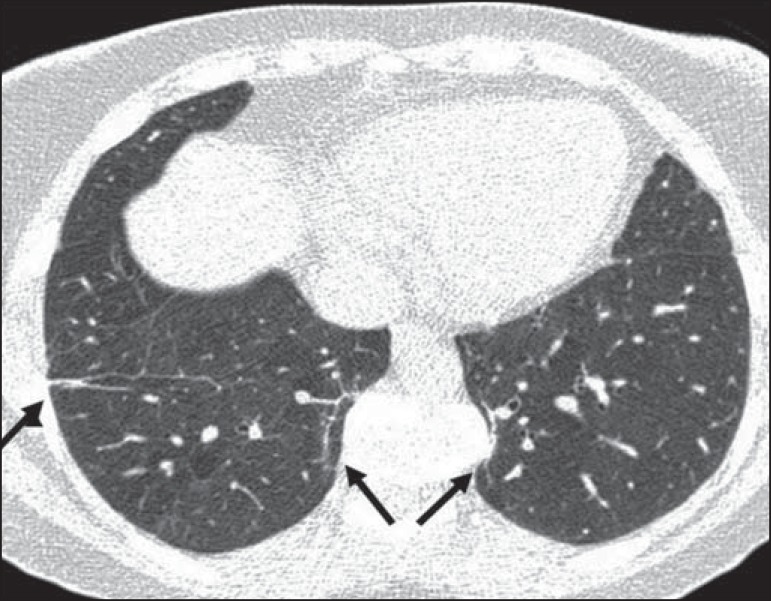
Chest CT scan of a patient with sickle cell disease, showing reticular
